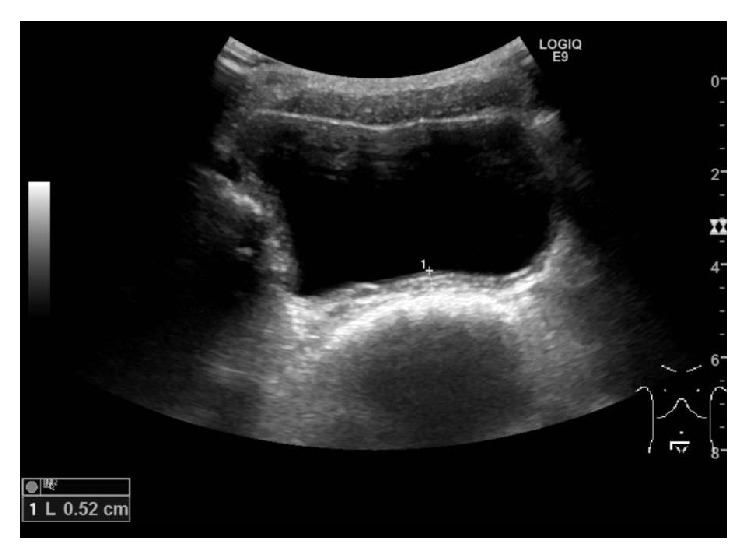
Ultrasound at clinical recurrence showed thickening of the bladder wall of up to 5 mm.
